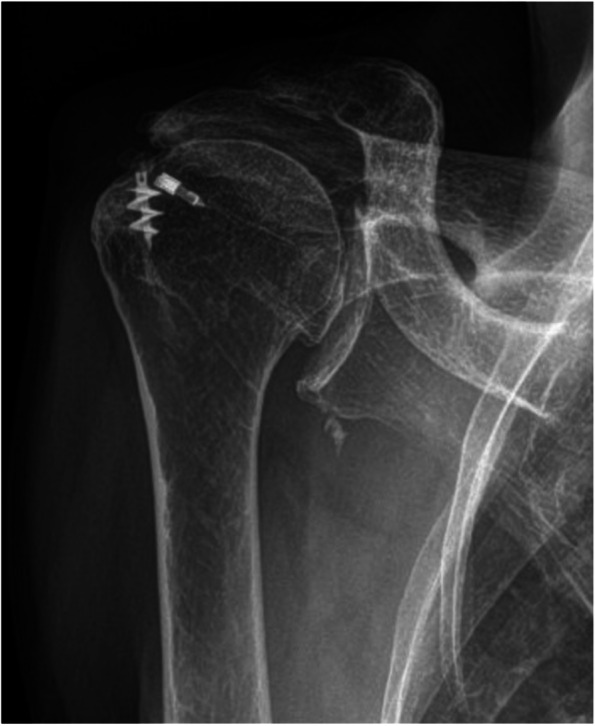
Preoperative grashey radiograph of the right shoulder demonstration superior migration of the humeral head with prior metallic anchor from a rotator cuff repair. The radiograph is consistent with rotator cuff tear arthropathy
